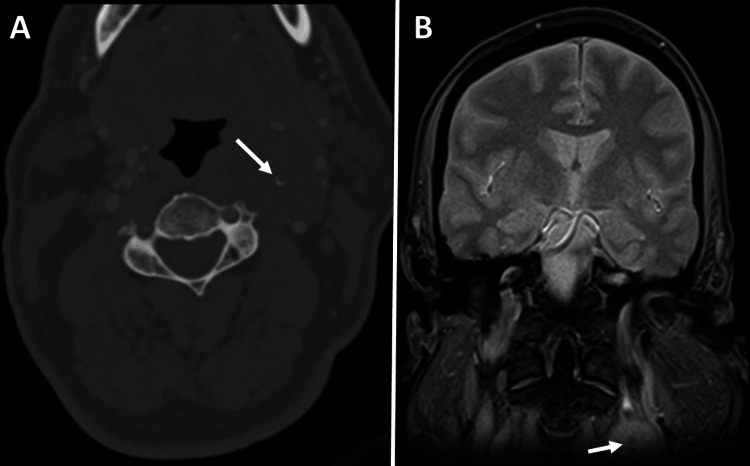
(A) Axial CTA of the neck in bone window setting demonstrates central punctate calcification (white arrow in A). (B) The coronal GRE image of the brain demonstrates partially included low signal intensity focus (white arrow in B) in the partially imaged lesion of left carotid space, which corresponds to the calcification seen in the CT scan. CTA: Computed tomography angiography; GRE: Gradient echo sequences.
